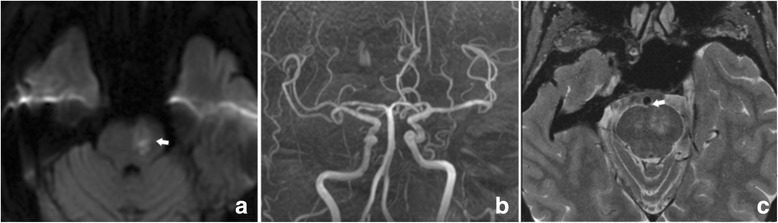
In a patient (60-69 year old) with acute isolated pontine infarction, (a) DWI showed paramedian infarct in the left pons (arrow); (b) MRA showed no obvious stenosis in the basilar artery; (c) T2 dark fluid SPAIR showed an atheromatous leison in the orifice of a paramedian artery (arrow)
